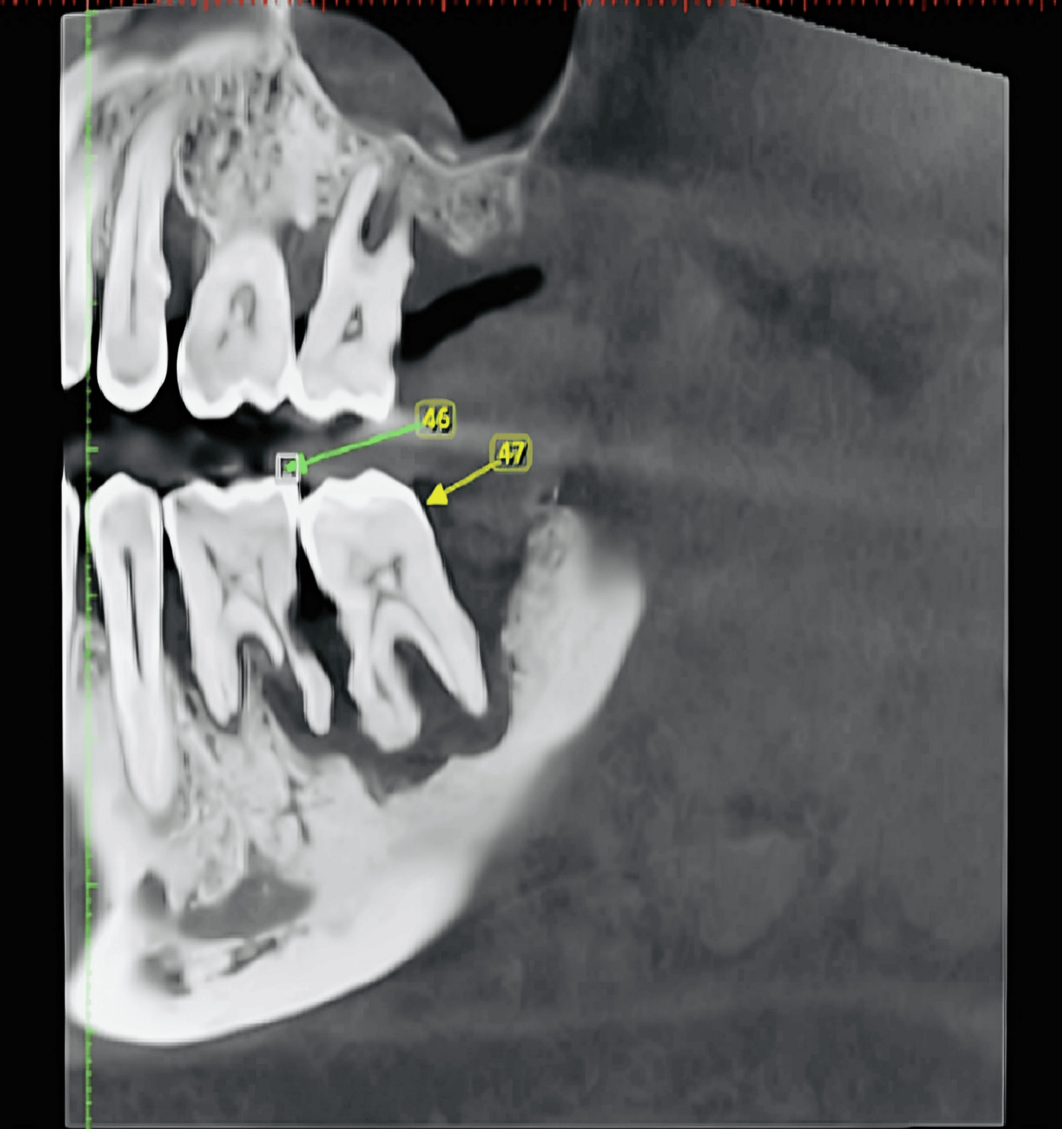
Sagittal view of CBCT revealing severe alveolar bone resorption and periapical rarefaction with 46, 47 CBCT: cone beam computed tomography
